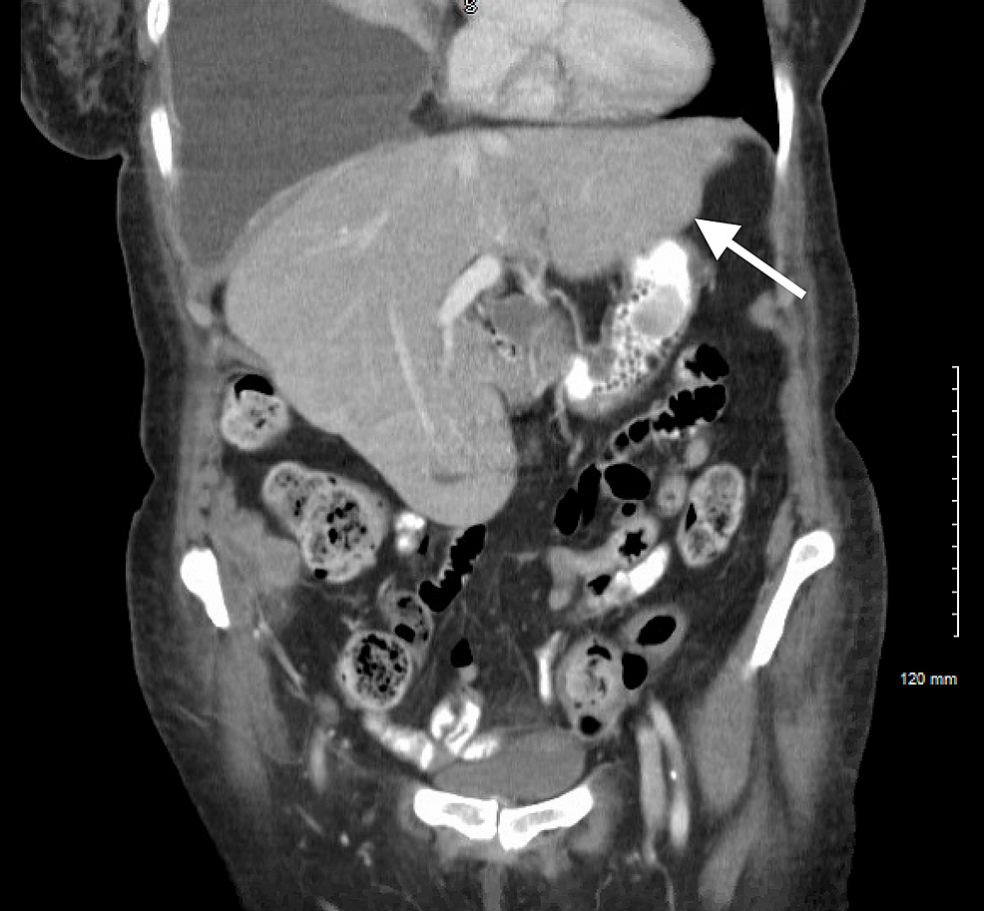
CT abdomen with left hepatic lobe lesion
